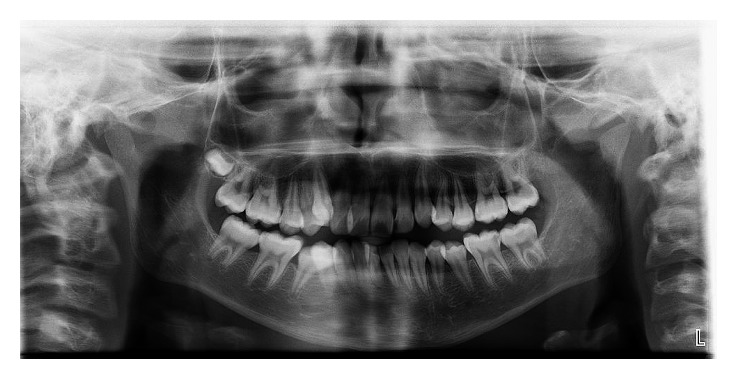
Panoramic radiograph at T0.
